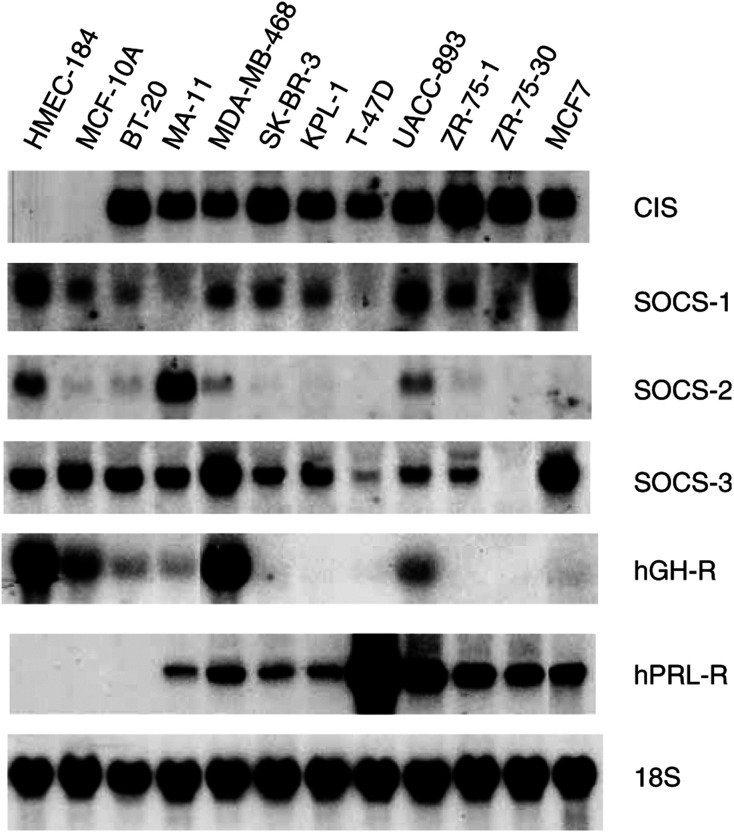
Expression of transcripts for CIS and SOCS-1–3 and for GH and prolactin receptors in normal (HMEC184 and MCF-10A) and 10 breast cancer lines. Total RNA was extracted from cell lines, subjected to electrophoresis on a formaldehyde gel and blotted on a nylon membrane. Identical blots were run and each was hybridized with a specific cDNA probe. The blots were then washed and exposed to X-ray films as described in Materials and Methods. The exposure time for CIS was 2 days; for SOCS-1, 5 days; for SOCS-2, 5 days; and for SOCS-3, 3 days. Blots were stripped and reprobed for 18S rRNA to ensure equal loading.
